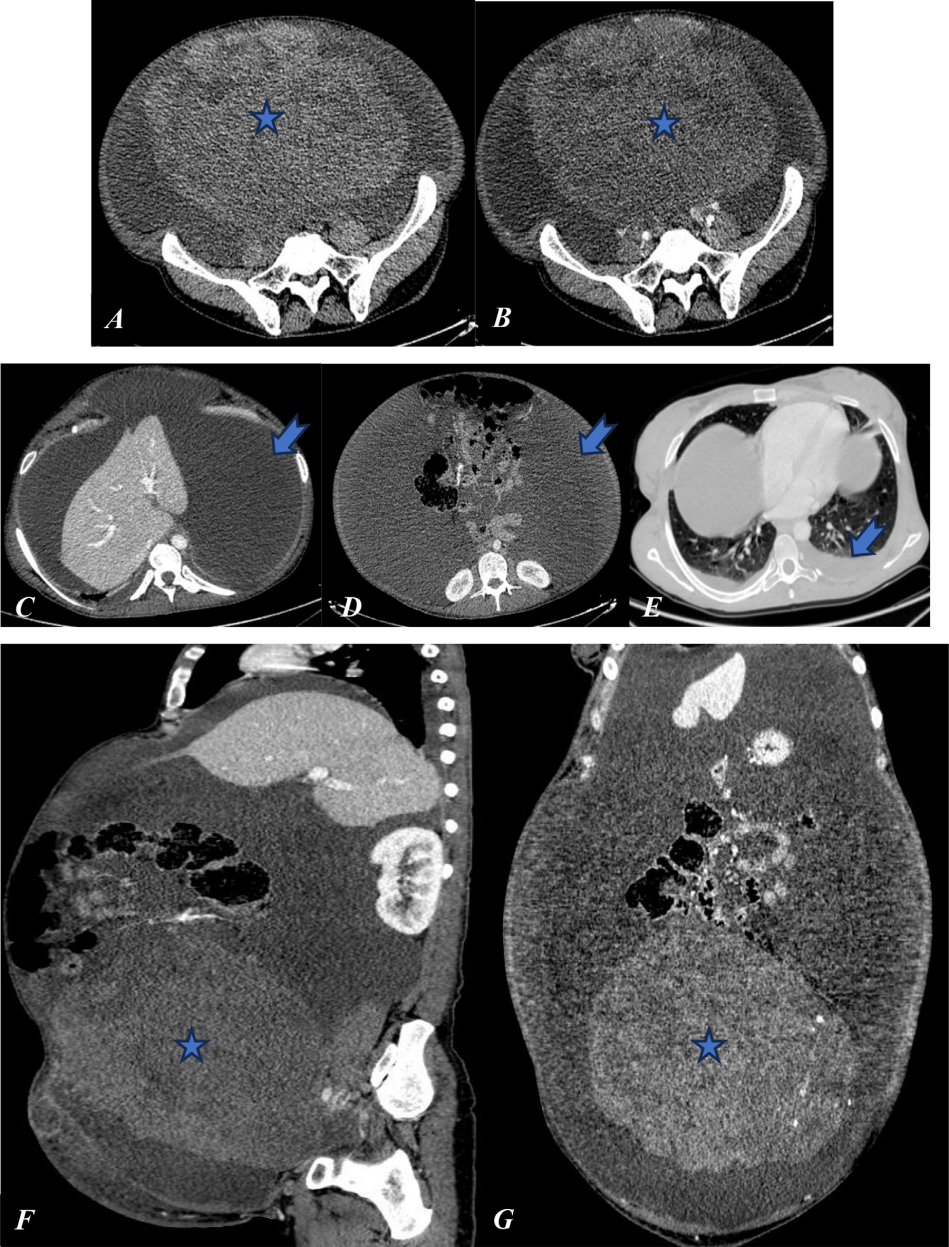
Axial (A, B), sagittal (F), and coronal (G) CT scans showing a large right ovarian mass (blue star). Axial CT scans showing intraperitoneal effusion (C, D) and pleural effusion (E) (blue arrow).
